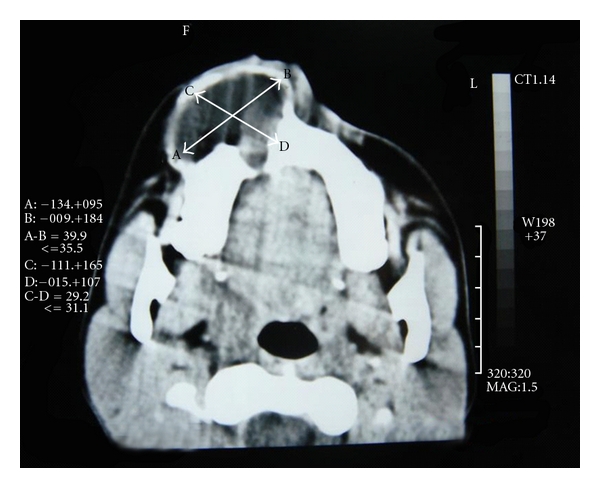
CT showing large expansile lytic lesion arising from the right maxilla extending into the right maxillary antrum and anterior portion of the right nasal cavity.
